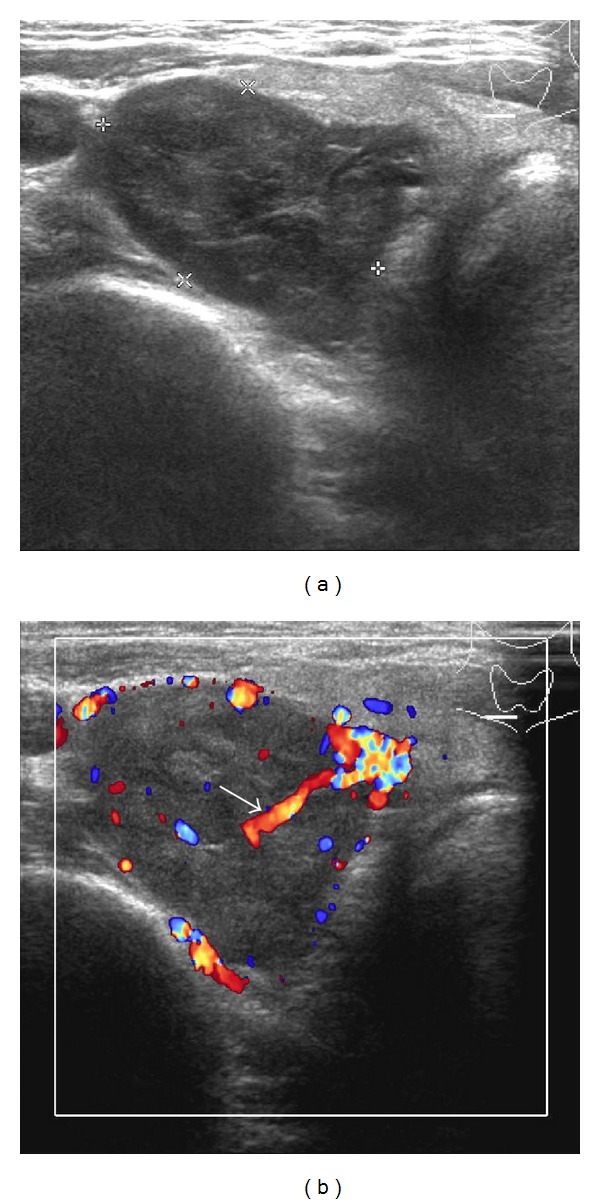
Ultrasonogram of thyroid gland and parathyroid glands. (a) Ultrasonogram and (b) color Doppler flow of right thyroid lobe showed a 2.8 × 1.9 cm heterogenous hypo-to-isoechoic with central hypervascularity solid mass located just inferior to right lobe of thyroid gland. The presence of extrathyroidal feeding artery or polar artery was shown (arrow). This mass was corresponding to palpable neck mass.
